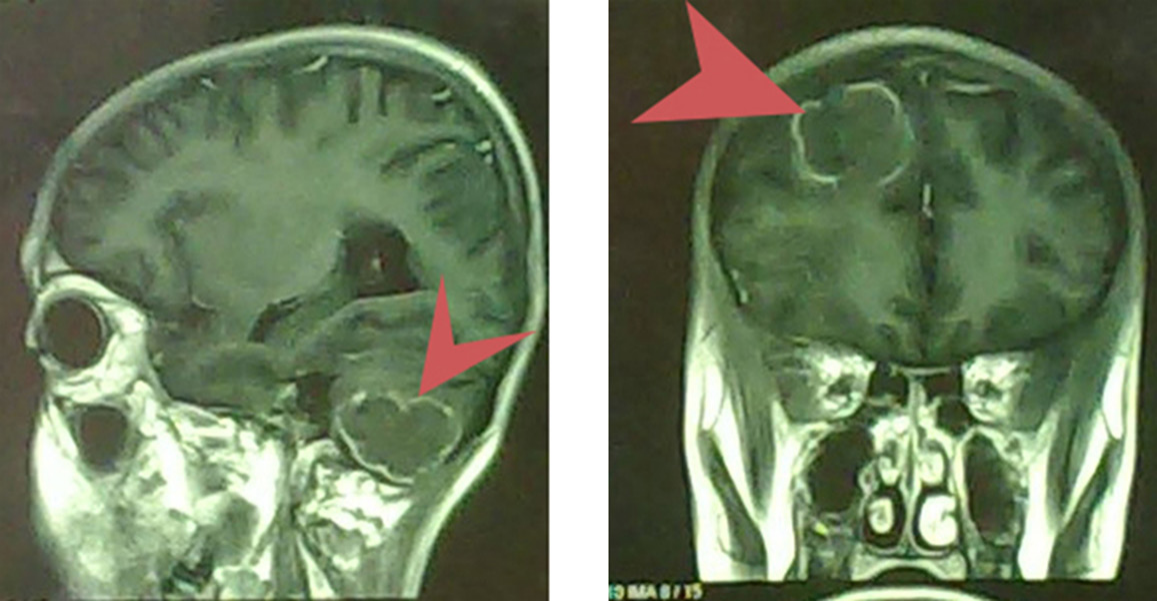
The magnetic resonance image of the brain showing enhancing nodules in deep white matter and subcortical area in the right fronto-parieto-temporal region and also left cerebellar hemisphere showing foci of hemorrhage with surrounding edema and mass effect and irregularity ring enhancement
